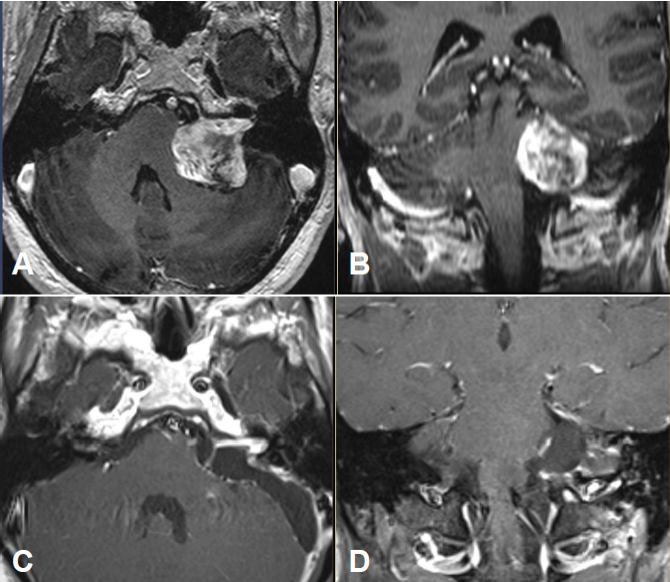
Preoperative MRI, T1-w + CM; A transversal; B coronal. 2 years after surgery, MRI T1-w + CM; C transversal; D coronal; liquor pad in the surgical pathway, linear homogeneous contrast enhancement in the left internal auditory meatus corresponding to the residual tumor.
